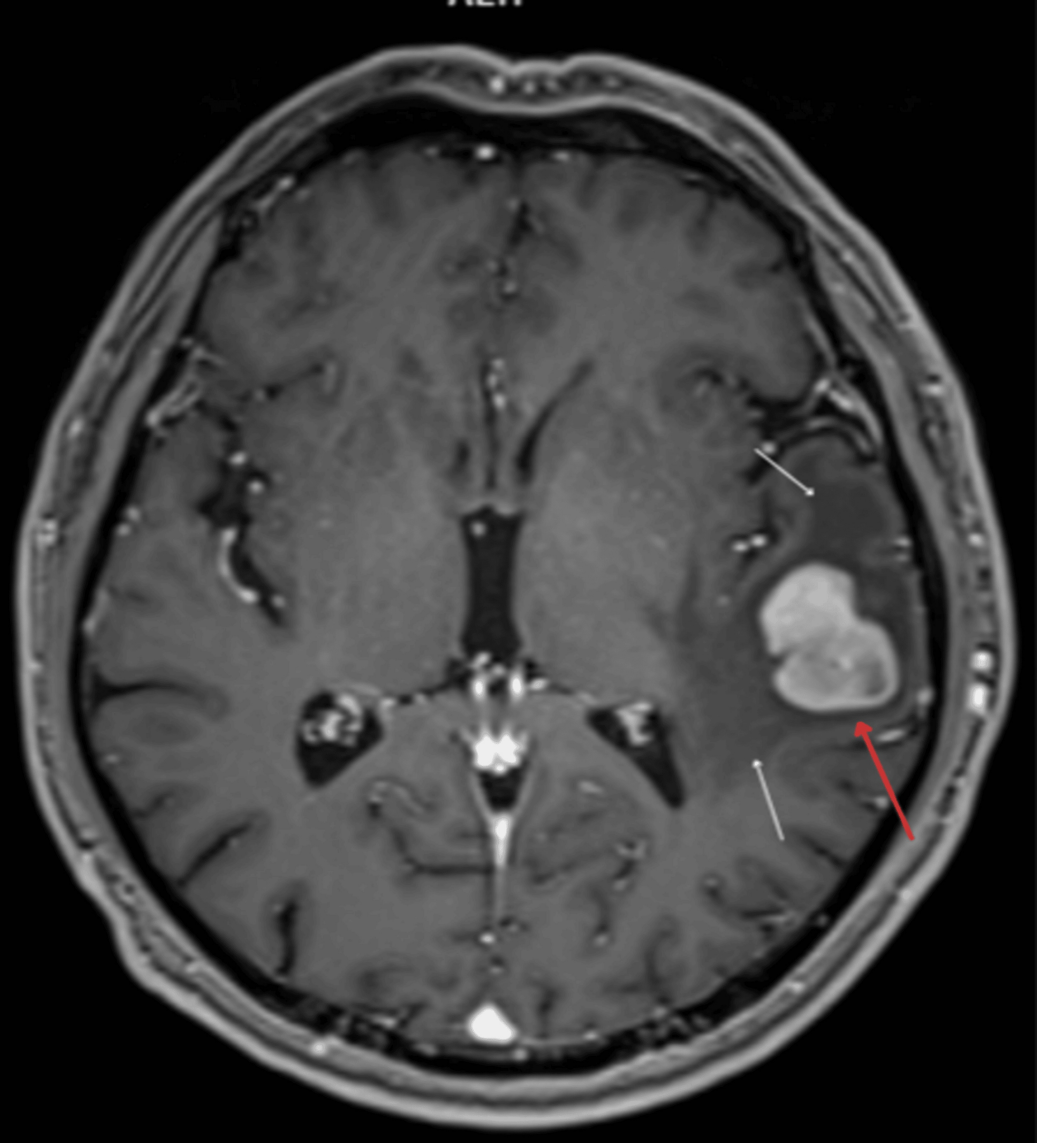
Brain magnetic resonance imaging (MRI). In the left temporal lobe, an irregular hyperdense focal lesion (red arrow) measuring 27x20x25 mm (APxRLxCC) surrounded by a hypodense, finger-shaped zone of edema (white arrows).
